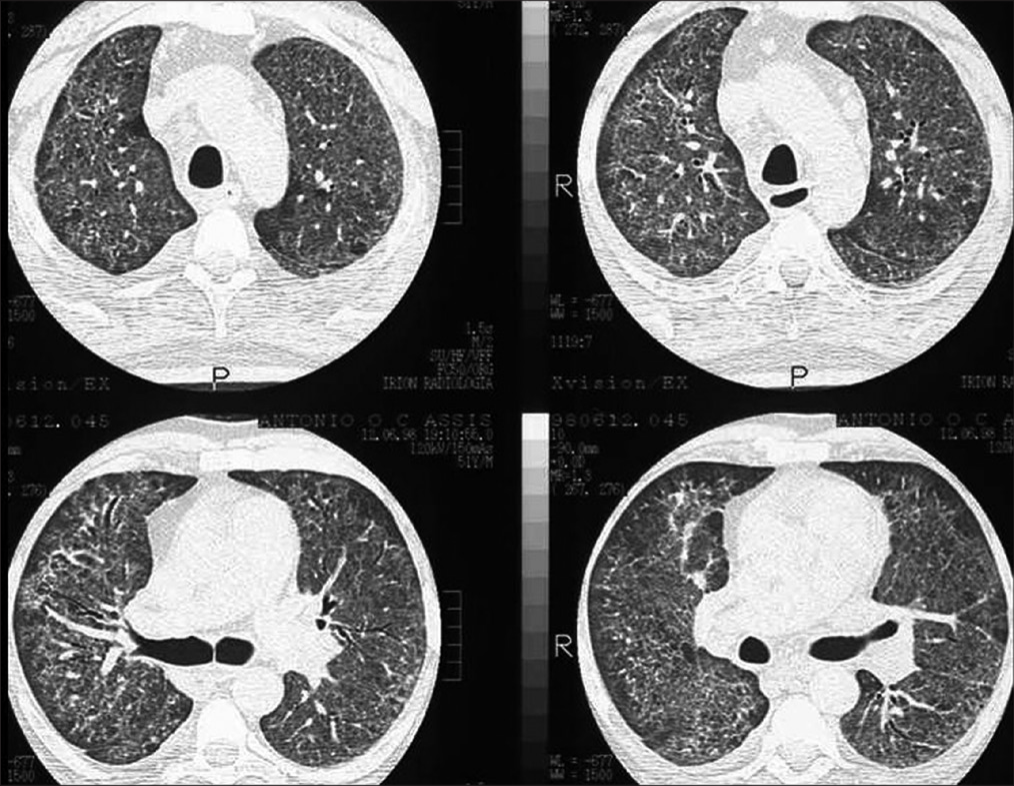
Pneumocystis carinii* pneumonia. High-resolution computed tomography shows diffuse ground-glass attenuation with a geographic distribution
